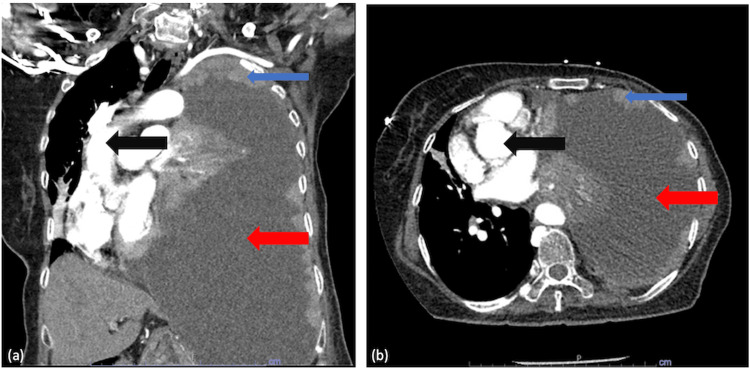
Computed tomography of the chest. (a) Coronal view and (b) axial view. A large pleural effusion (red arrow) with complete atelectasis of the left lung with dextroposition of the heart and mediastinal structures (black arrows) to the right. There is also significant pleural nodularity (blue arrows).
